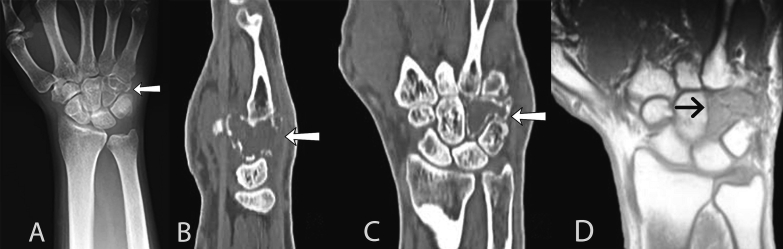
(a) Preoperative anteroposterior radiograph of the wrist showing expansile lytic lesion of the hamate. (b) Coronal and (c) sagittal sections of computed tomography show a lytic lesion at the hamate bone, expansile, loss of the intraosseous trabecular pattern, and cortical disruption in the dorsal bone surface. (d) The coronal section of T1-weighted MRI confirmed tumor extension to the distal one-third of the triquetum bone showing the other carpal bones unaltered.
